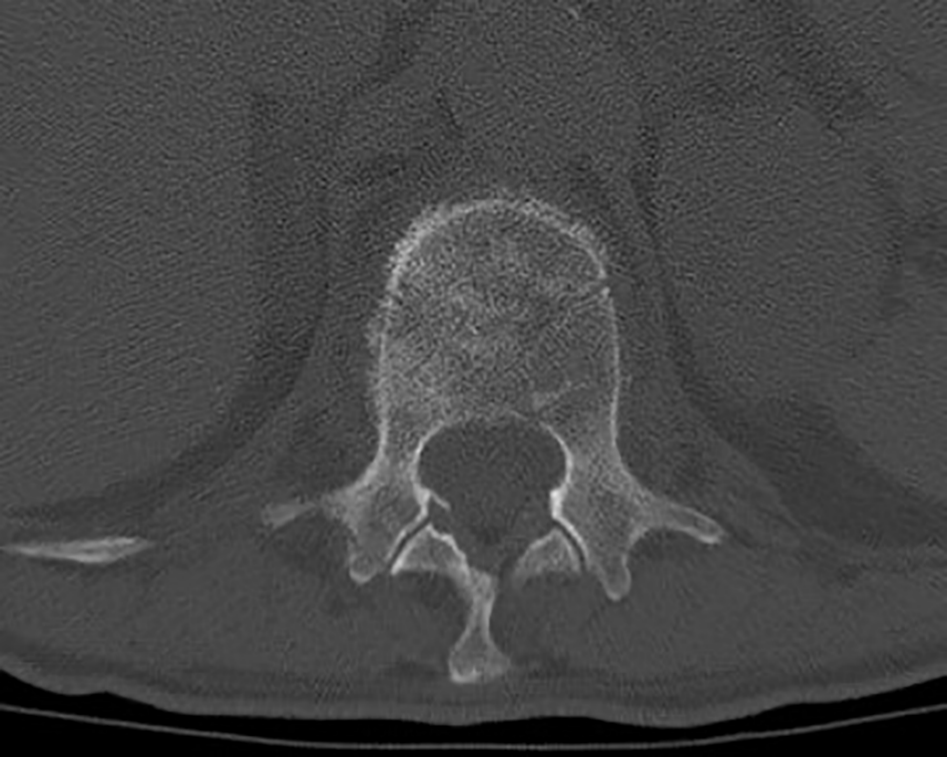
Axial thoraco-lumbar CT scan showing L1 metastatic lesion with altered bone density and osteolytic areas.
